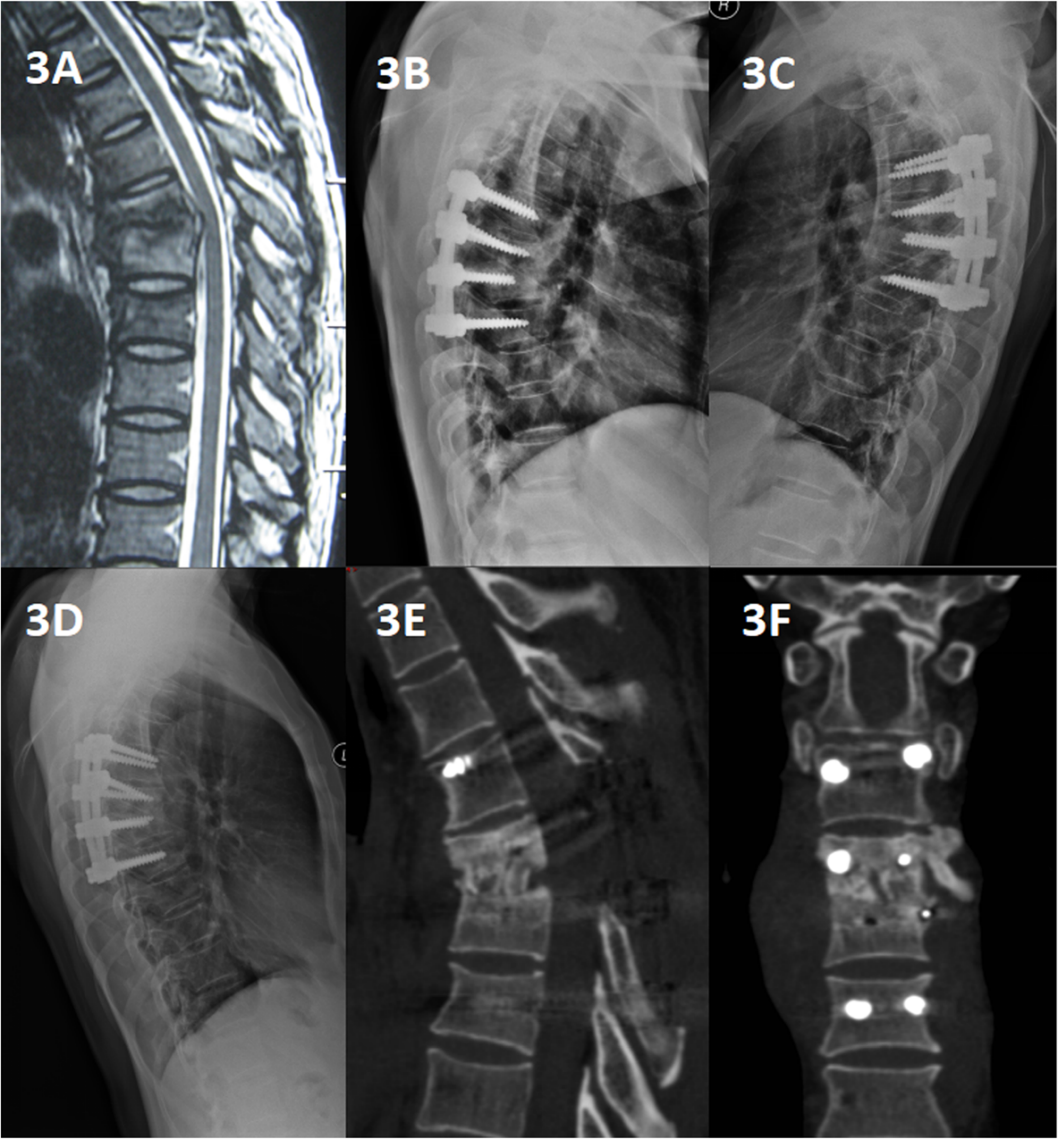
An 18-year-old man with thoracic spinal tuberculosis (T6-7) underwentsingle-segment posterior debridement and decompression combined with internal fixation. (a) Pretreatment MRI showing destruction of the T6-7 vertebrae and concomitant compression of the spinal cord. (b,c) Immediate postoperative and 6-months radiographs demonstrating posterior debridement, bone graft and internal fixation. (d,e,f) At the 36-month follow-up, plain Xray and CT showed maintenance of the correction and solid fusion
